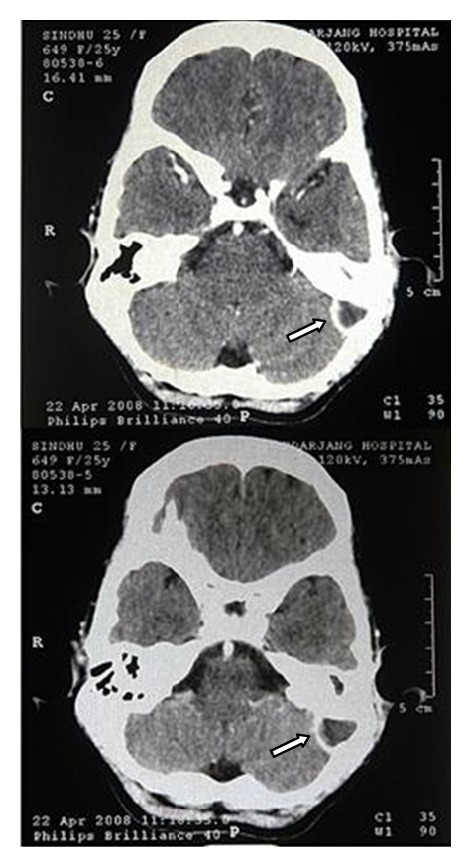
CT scan showing lateral sinus thrombosis.
